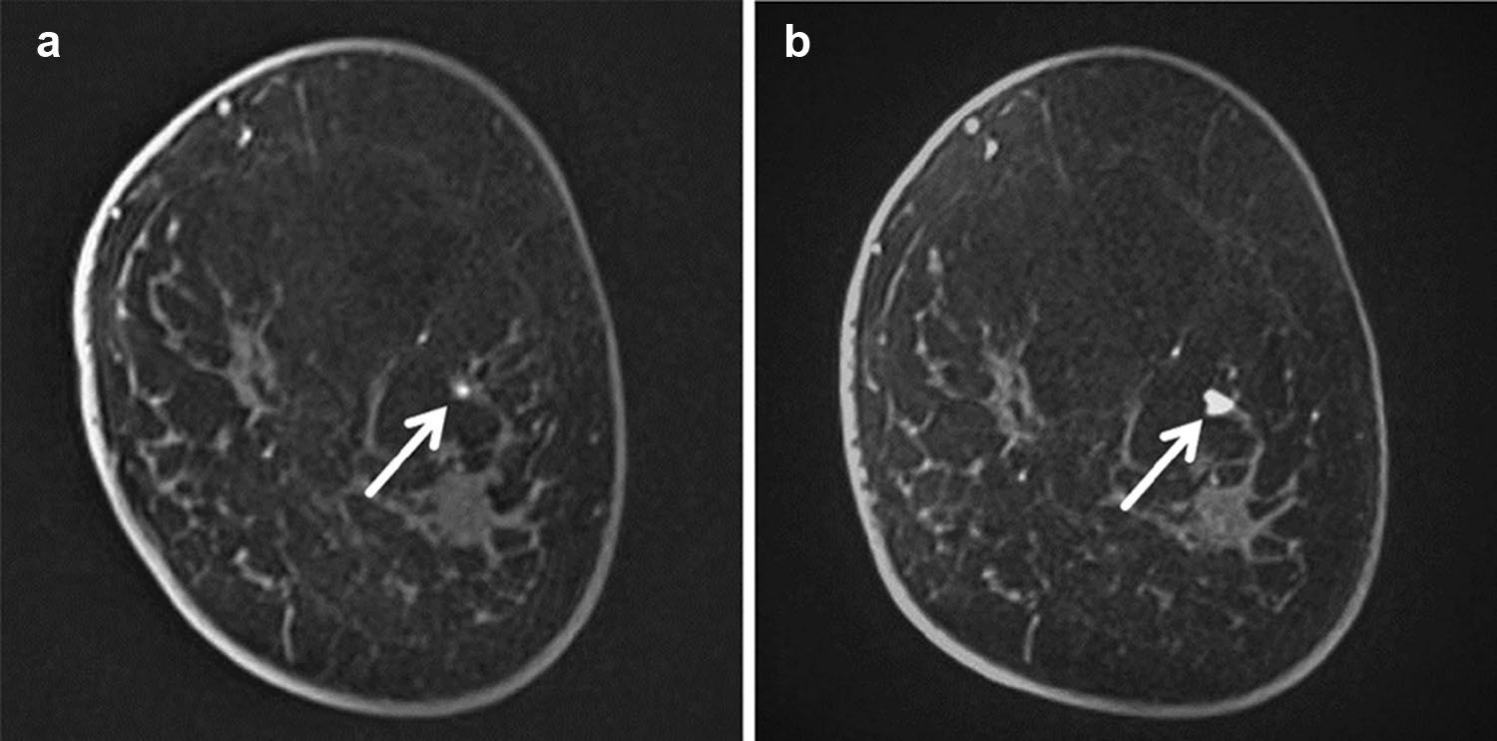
a, b Coronal first dynamic contrast-enhanced MR image of left breast in 35-year-old female BRCA1 mutation carrier. These MR images were performed for post-operative contralateral follow-up as she underwent surgery for the right breast at the age of 32. A new focus lesion was detected in 3 mm in diameter on MR surveillance (arrow of a). After observation for 6 months, it increased to 7 mm in diameter (arrow of b). As this lesion could not be detected on second-look ultrasound, MRI-guided biopsy was performed. It was diagnosed as triple-negative ductal carcinoma in situ (DCIS)
